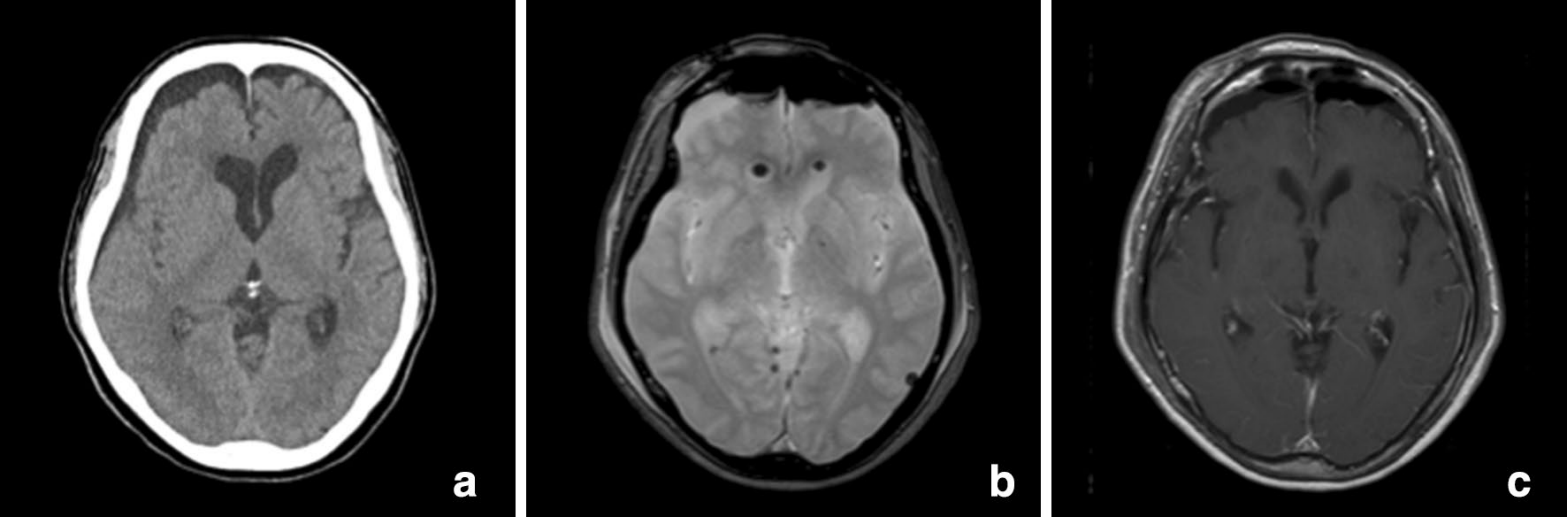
Postoperative imaging showed no evidence of residual mass, and demonstrated improvement of hydrocephalus. a Postoperative computed tomography image, b T2-star-weighted image, and c gadolinium-enhanced T1-weighted image
